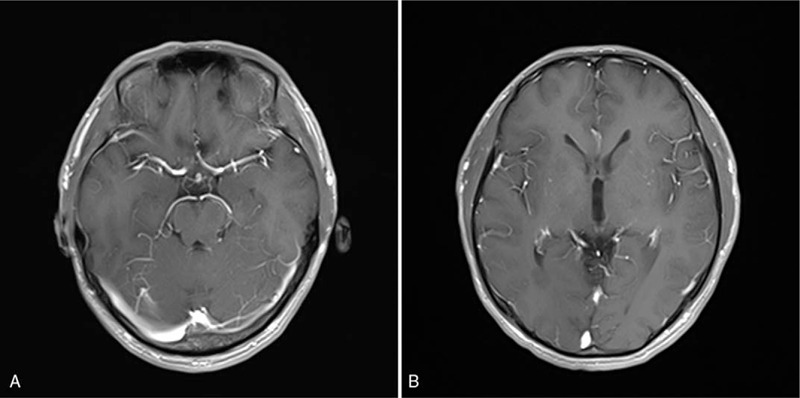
Brain T1-weighted postcontrast MRI of anti-IgLON5 disease. Representative axial, T1-weighted postcontrast MRI demonstrating no significant postcontrast enhancement of left tegmentum of the midbrain (A) and right occipital horn of the lateral ventricle (B).
